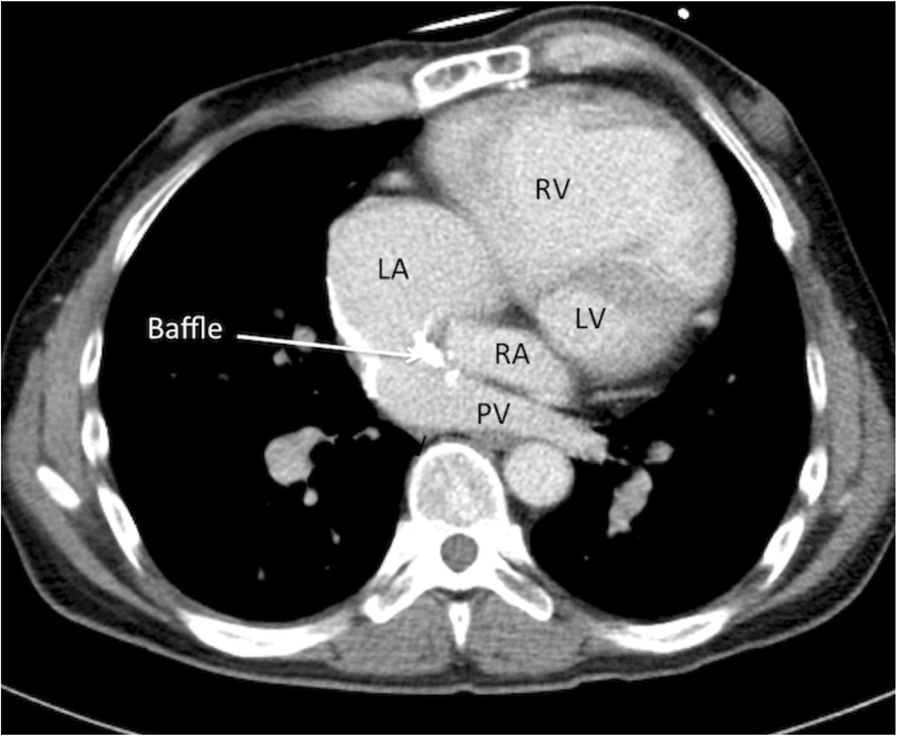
Computed tomography just before systemic ventricular assist device implantation shows baffle calcification, a slightly narrowed pulmonary vein, and dilated left atrium and right ventricle. *LA* left atrium, *RV* right ventricle, *RA* right atrium, *LV* left ventricle, *PV* pulmonary vein
